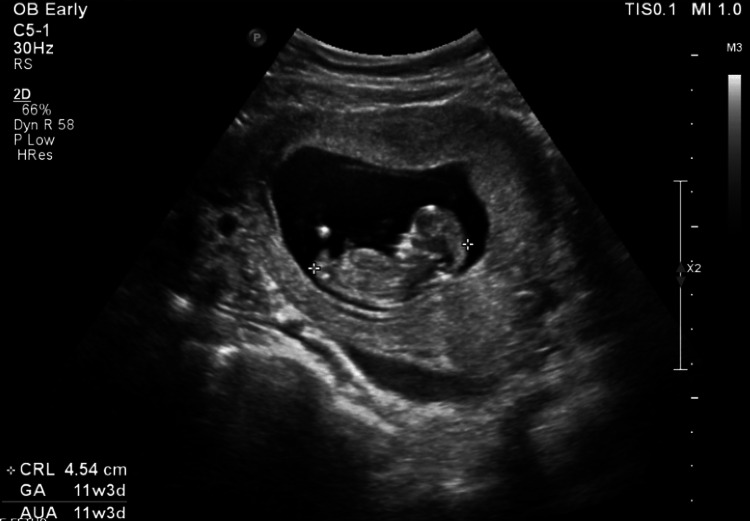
Transabdominal ultrasound demonstrating a live intrauterine pregnancy with fetal cardiac activity.
